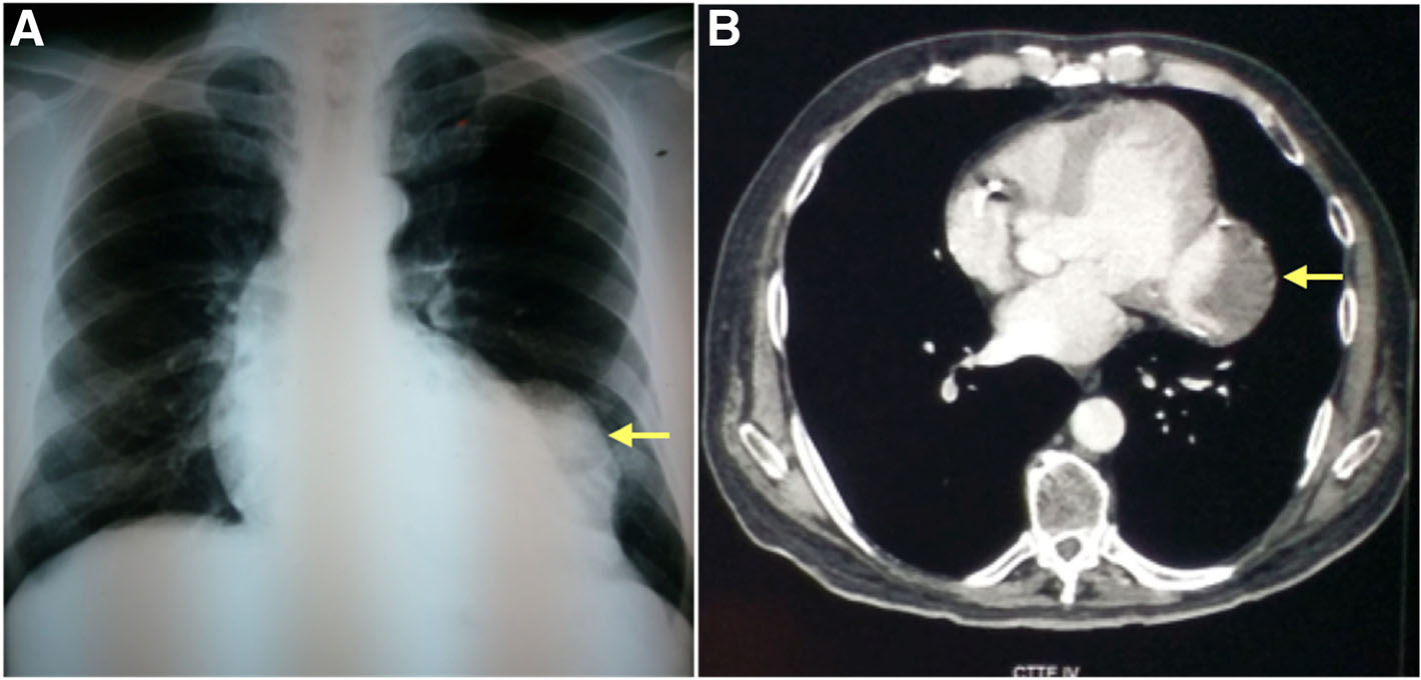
a Chest x-ray showing mild cardiomegaly, of note, a homogeneous opacity adjacent to the LV (arrow). b Chest CT showing a saccular image in the posterolateral wall of the LV that corresponds to a thrombosed PSA in the LV free -wall (arrow)
